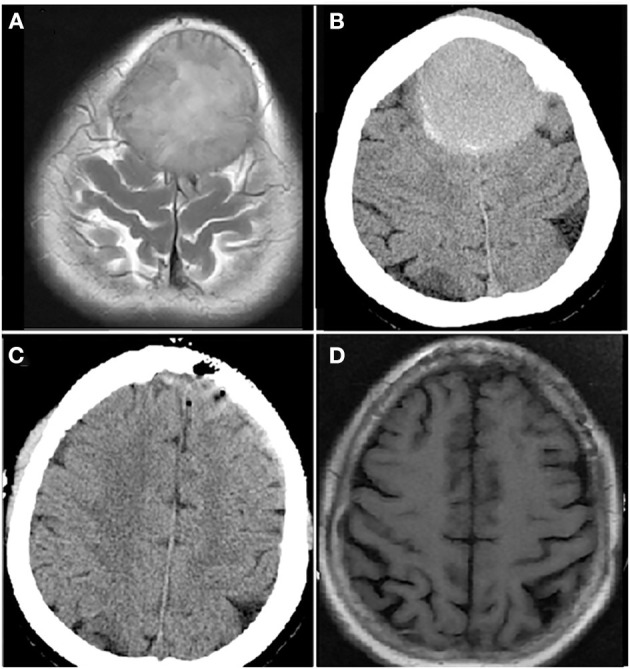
Computer tomography (CT) and magnetic resonance imaging (MRI) brain images from a female patient present with bilateral frontal mass. Enhanced MRI brain image (A) and CT brain image (B) at diagnosis showed a mass of 5.3 × 7.0 cm in bilateral frontal lobe; CT brain image after resection of bilateral frontal lobe mass, the mass disappeared (C); MRI brain image before autologous hematopoietic stem cell transplantation (auto-HSCT), no mass in bilateral frontal lobe (D).
